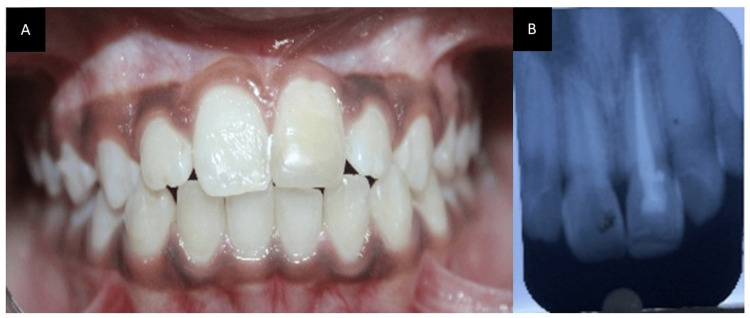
Post-extrusion status and endodontic outcome. A) Intraoral frontal view after completion of orthodontic extrusion, showing satisfactory alignment of tooth 21, with an acceptable gingival contour and aesthetics. B) Periapical radiograph confirming root canal obturation, re-establishment of the periodontal ligament space, and absence of pathological root resorption or periapical pathology.
